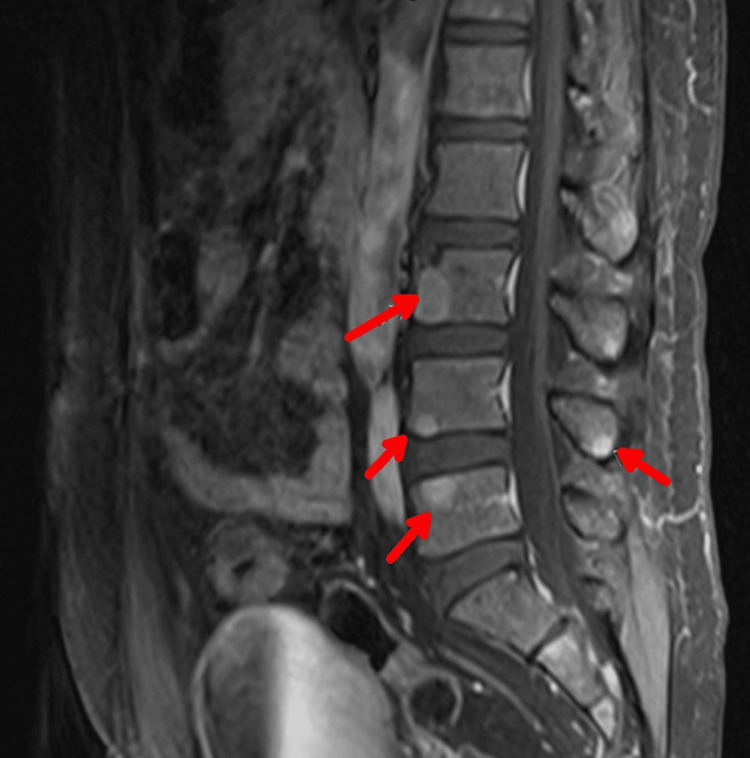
MRI (magnetic resonance imaging) lumbar spine with contrast showing widespread osseous enhancing lesions (red arrows). Osseous lesions can be seen in many conditions, but in conjunction with the patient’s initial lab values they are concerning for MM.
